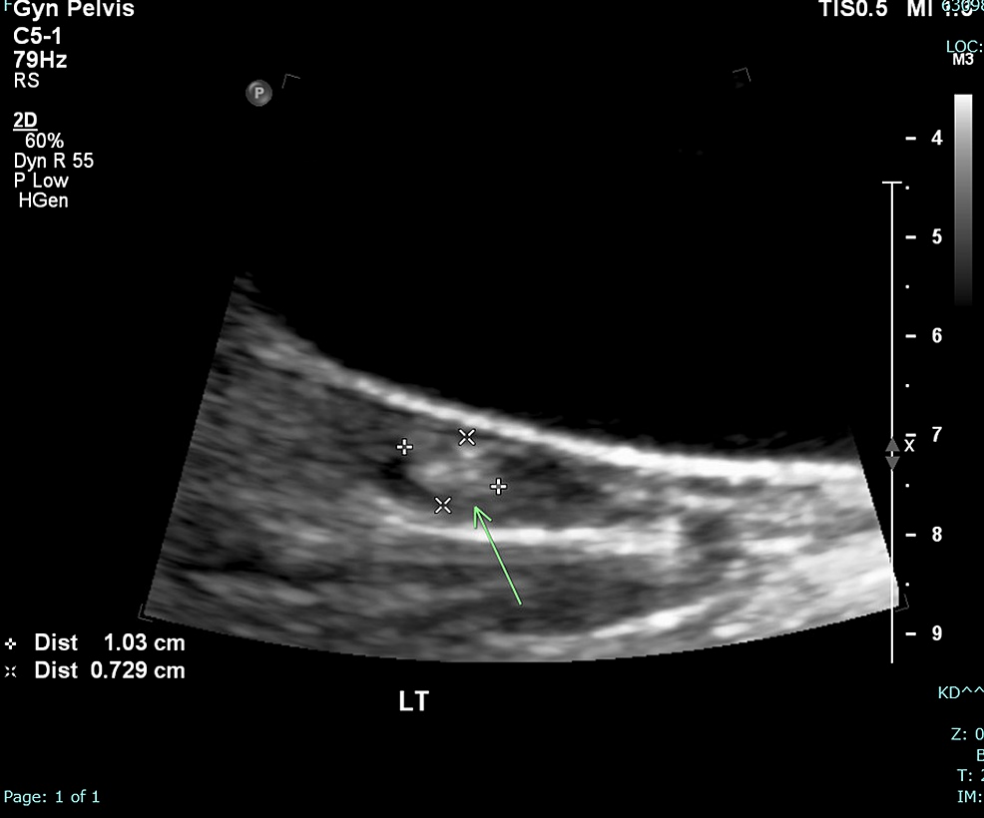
Nonobstetric pelvic ultrasound showing 1 cm hyperechoic left ovarian lesion.
